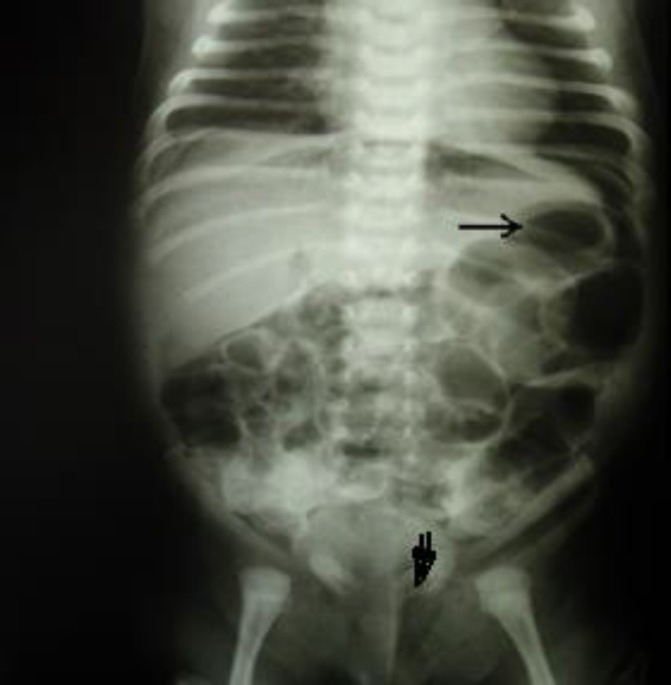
Abdominal x-ray shows free intraperitoneal gas and Rigler sign (gas in the inner and outer side of the bowel loop- arrow).The open arrow shows gas in the left scrotal sac.
